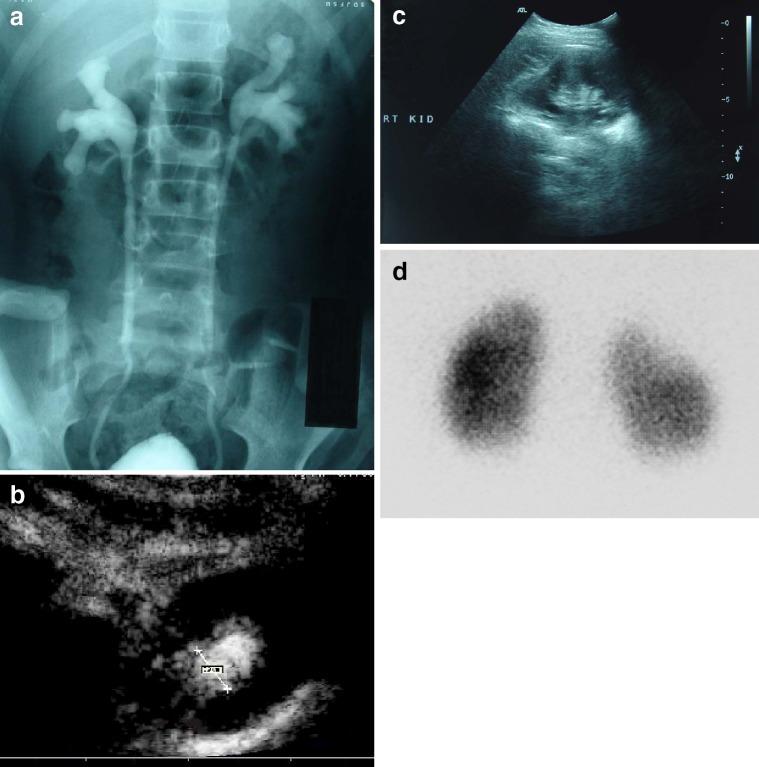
Radiological imaging of a 7-year-old girl with a history of acute pyelonephritis. a Voiding cystourethrography shows bilateral vesicoureteral reflux (VUR) grade III. b Contrast-enhanced harmonic voiding urosonography (VUS) (transverse section in prone position) shows also VUR grade III. The same image was found in VUS on the left kidney (not shown). c Ultrasound of the right kidney reveals irregularity of renal outline and focal thinning of renal cortex in the upper pole, findings compatible with scar. d Posterior view of 99mTc-dimercaptosuccinic acid (DMSA) scan 6 months after an acute pyelonephritis shows a focal defect in radiotracer uptake of the upper pole on the right kidney, indicating the presence of a renal scar
